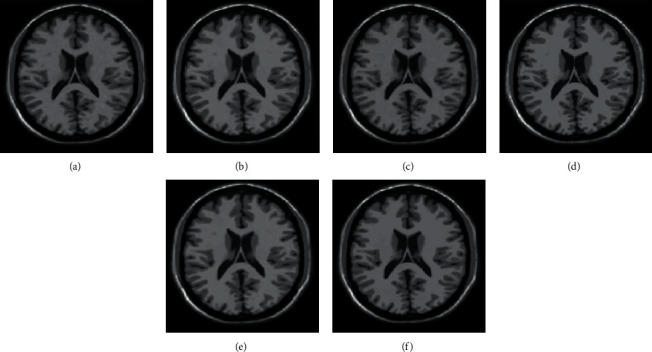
Various techniques are comparatively analyzed by using the digital MR image. (a) Bilinear interpolation [44]. (b) BI with the prefilter [41]. (c) High-boost filter [7]. (d) APNN [42]. (e) UTDCF [43]. (f) Proposed EWMI.
